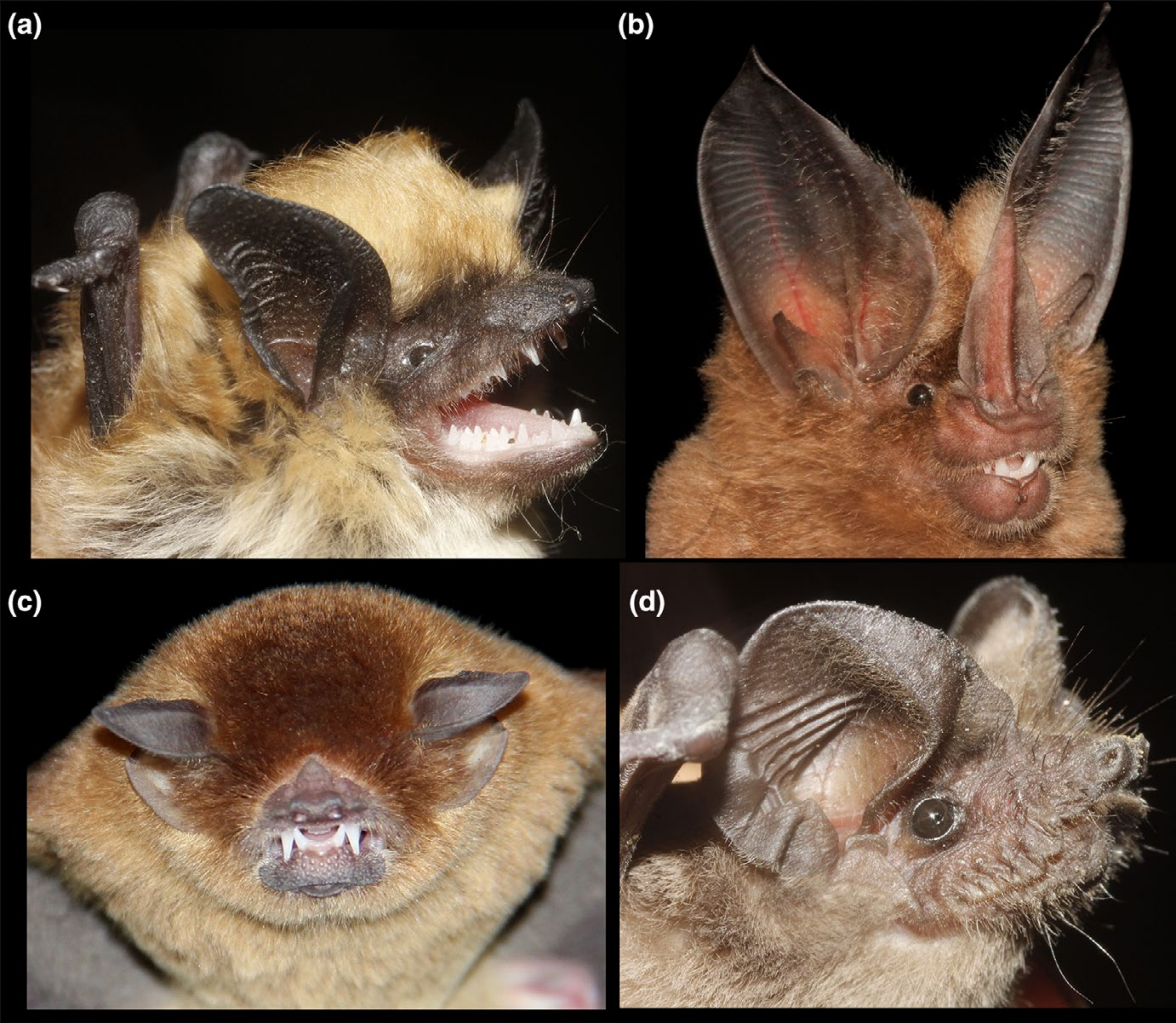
Mexican bats representative of different echolocation strategies. (a) *Myotis californicus* belongs to the Vespertilionidae family whose echolocation calls can be characterized by presenting broadband‐modulated frequencies, which are of relatively high intensity and short duration; (b) *Mimon cozumelae* is a member of the Phyllostomidae family and emits echolocation calls (usually of low intensity) composed of multiharmonic components and constant modulated frequencies; (c) *Pteronotus parnellii* emits typical calls of the Mormoopidae family consisting of a constant frequency segment, followed by a modulated sweep descendent call, and finalizing with a quasi‐constant frequency with a short duration, and it is the only species in America that compensates for the Doppler effect; (d) *Tadarida brasiliensis* is a representative of the Molossidae family with typical echolocation calls of open space foragers with relatively low frequencies and long call durations
